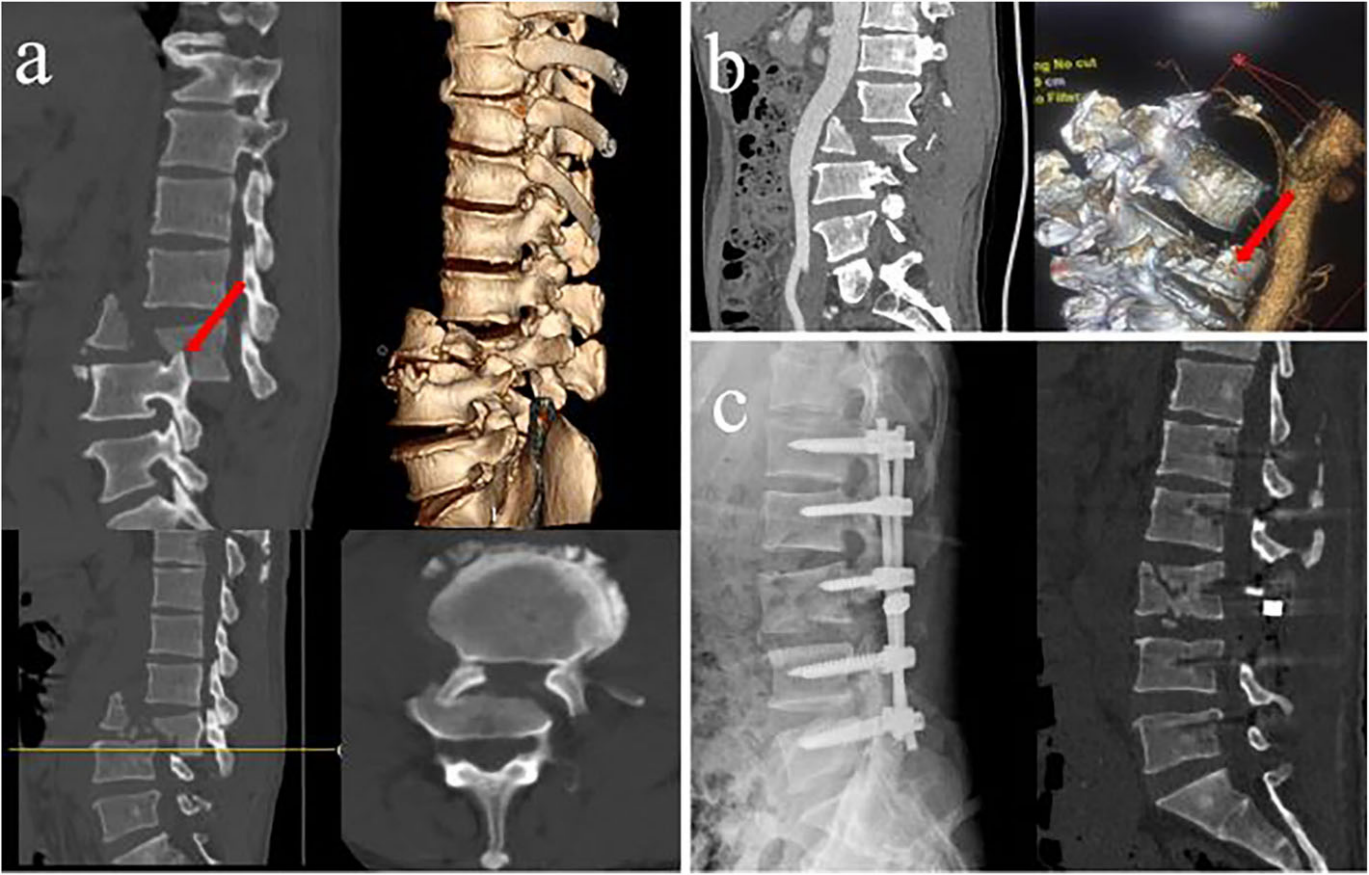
a Computed tomography (CT) showed L3 vertebral burst fracture with posterior dislocation, and fractured vertebral body formed articular process interlocking. b Computed tomography angiography (CTA) indicated fractured lumbar vertebra is adjacent to abdominal aorta and lumbar artery ruptured without active bleeding. c Postoperative X-ray and CT
